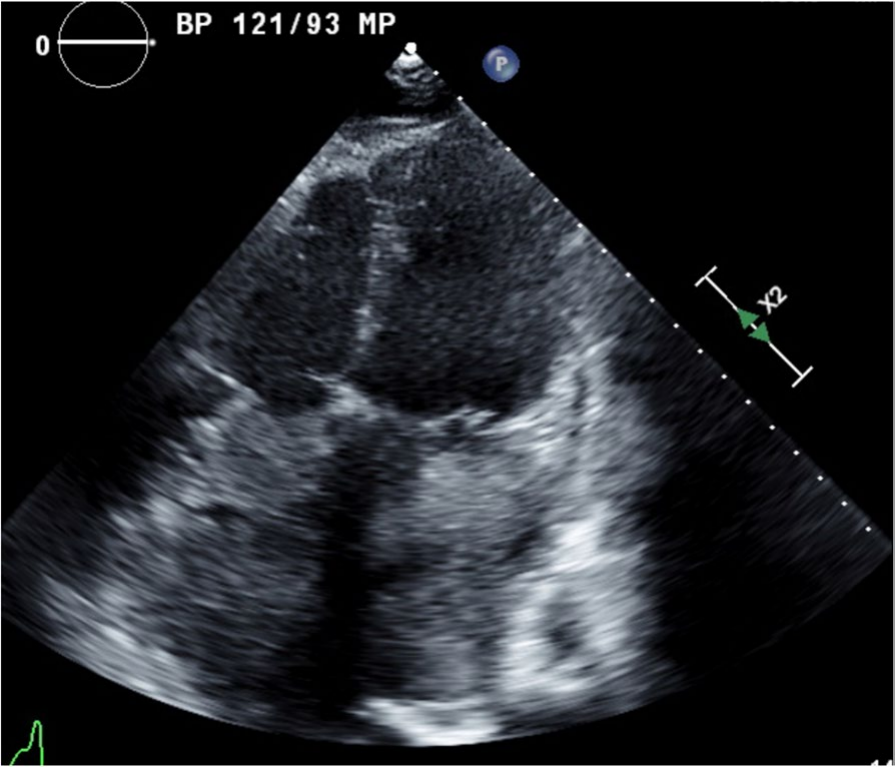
Echocardiogram with apical four-chamber view showing large atrial mass occupying both left atrium and right atrium, obliterating the interatrial septum
